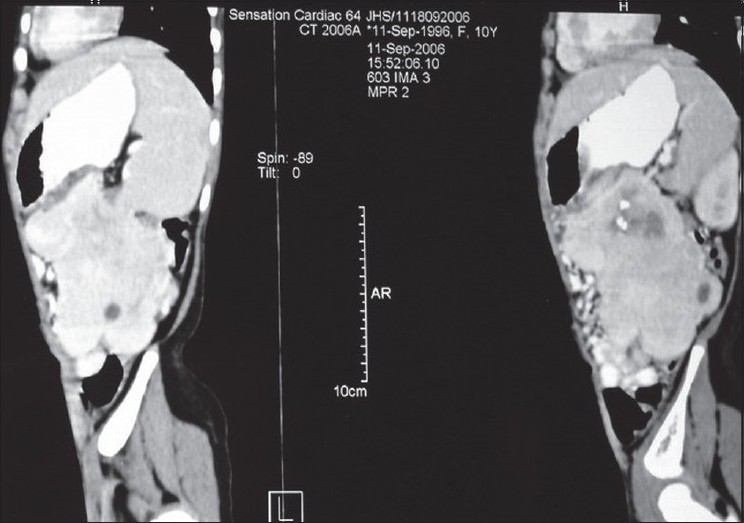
Computed tomography scan of the abdomen showing the large tumor inferior to the stomach and displacing the stomach and colon
